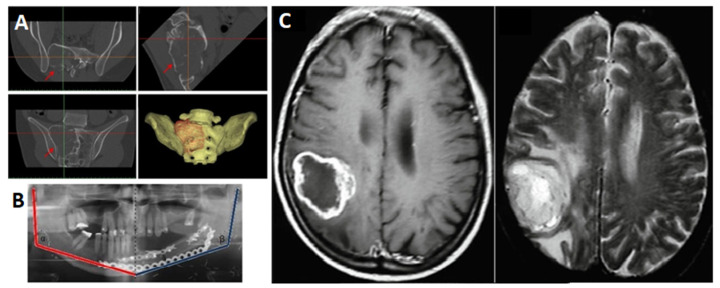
Examples of different use of medical imaging: (A) CT images of the pelvis and the generated 3D model [28], (B) measurements taken on X-ray medical imaging after mandibular reconstruction surgery [29], (C) MRI of the brain [30].
